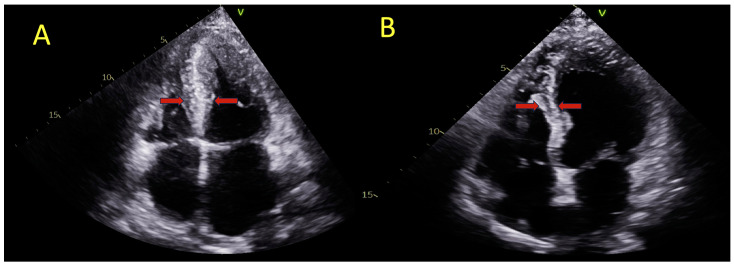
Two-dimensional echocardiographic four-chamber view at end-diastole depicting a patient with apical hypertrophic cardiomyopathy and septal involvement (A), and a patient with pure apical hypertrophy (B). The red arrows highlight the interventricular septal thickness.
